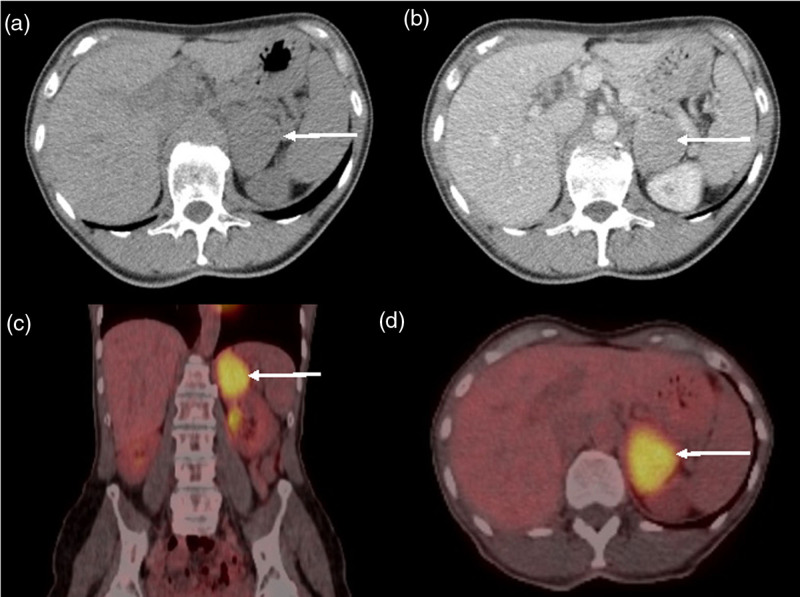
Preoperative computed tomography (CT) scans. Noncontrast CT (a) of left adrenal mass (white arrow) shows a mean attenuation of 43 HU with enhancement on the portal venous phase (b) with mean attenuation of 88 HU. Coronal (c) and axial (d) F-18 fluorodeoxyglucose (FDG) PET findings show significant tracer uptake in the left adrenal mass, with a maximum standardised uptake value (SUVmax) of 15, and adrenal tumour to liver SUVmax ratio of 5.
